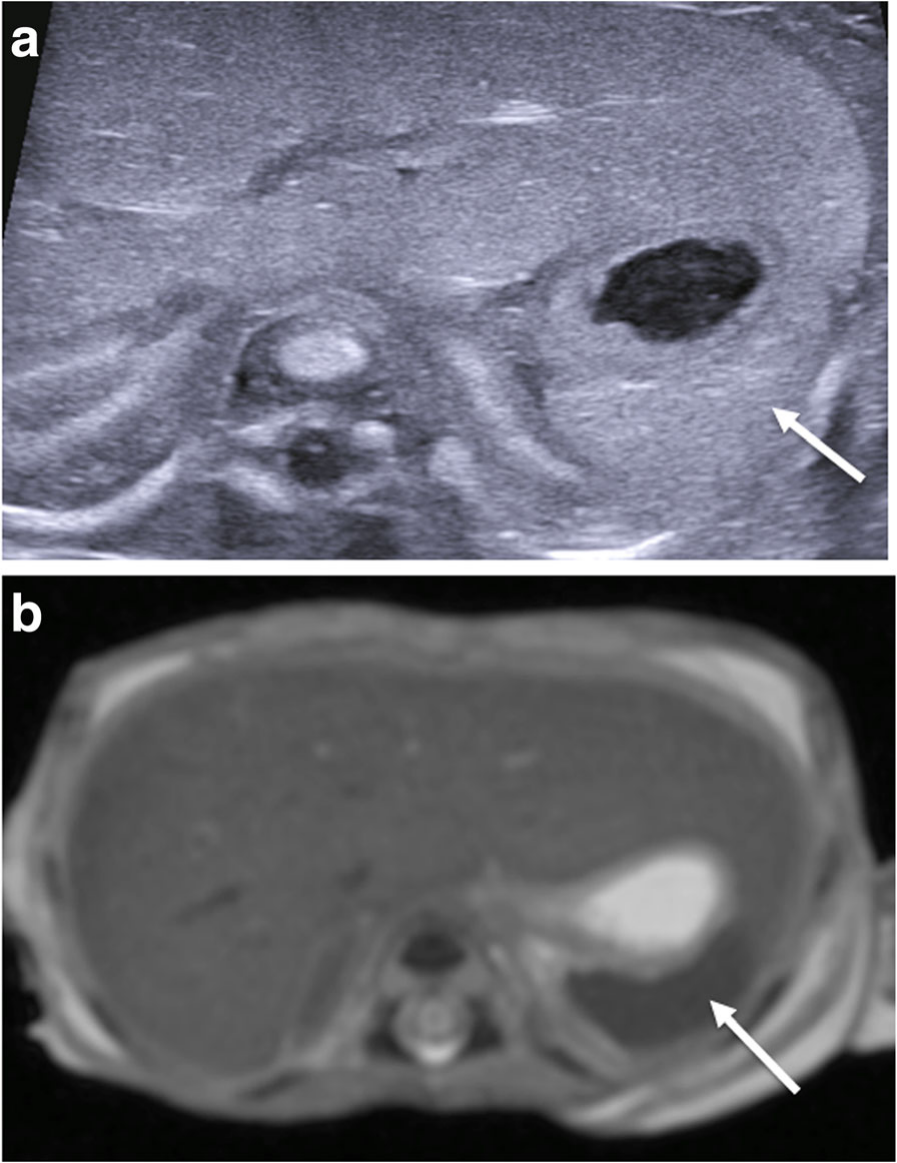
Normal appearances of the liver and spleen in a 19-week gestational-aged foetus following miscarriage, imaged 6 days after death. The transverse postmortem ultrasound imaging (a) through the upper abdomen demonstrates a very similar echogenicity of the liver with the spleen (white arrow). The differences between the two organs is much better seen at T2-weighted postmortem MRI (b), where both organs demonstrate different signal intensities
